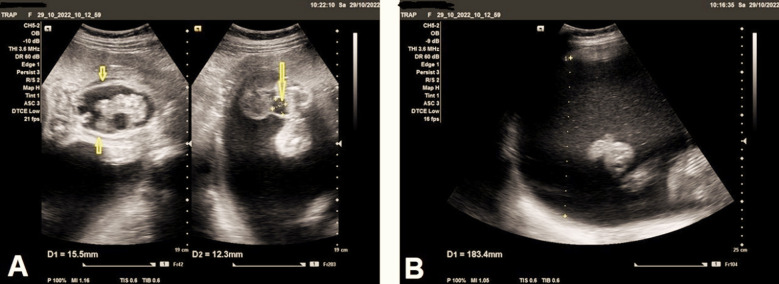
(A) Ultrasound imaging study of the acardiac twin which was stuck to the anterior uterine superiorly by amniotic band and shows markedly under-developed upper body organs including upper limbs, upper chest, non-developed head, and non-developed fetal heart. In addition to marked ascites, and edematous changes of the fetal body (signs of fetal hydrops), (short arrows), and cyst in the base of the umbilical cord of about 12mm in maximal dimension (long arrow). (B) Ultrasound imaging study shows markedly increased amniotic fluid volume, the largest pocket of approximately 183 mm, and amniotic fluid index (AFI) around 30 cm, indicating marked polyhydramnios.
